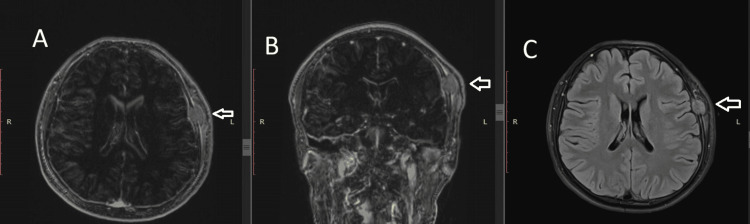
Preoperative brain MRI. (A) Axial T1-weighted post-contrast image demonstrating a moderately hypointense, partially circumscribed osteolytic lesion arising from the frontotemporal bone with intracranial extension, measuring 16 × 14 × 22 mm (AP × transverse × craniocaudal) (arrow). (B) Coronal T1-weighted post-contrast image showing the lesion's relationship to the frontotemporal bone, with osseous destruction and epidural extension (arrow). (C) Axial T2-FLAIR image revealing an isointense lesion without perilesional edema, consistent with an extra-axial compressive process without parenchymal invasion (arrow). FLAIR, fluid-attenuated inversion recovery
